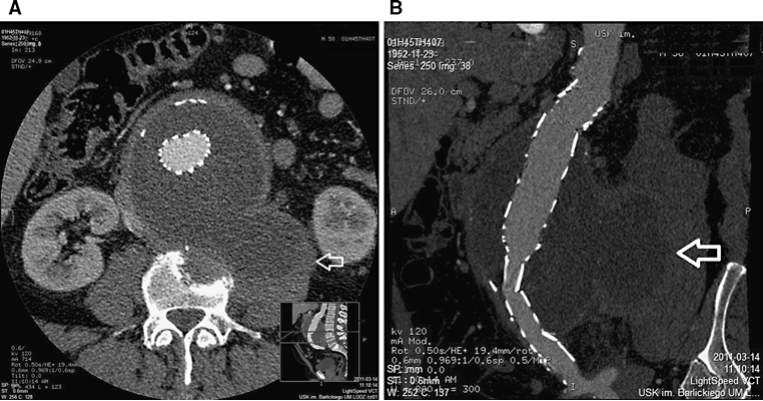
Angio-CT axial scan of aortic aneurysm below renal arteries. After implantation of the stent graft, patency is maintained. A Within the anterior and lateral walls neighboring the aorta, there is a soft tissue infiltration encompassing the inferior vena cava. A hematoma and inflammatory infiltration is probably present within the left iliopsoas muscle (*arrow*). Osteolysis of the L4 vertebral body is evident. B Reconstruction in the longitudinal plane confirms patency of the graft without leaking into the aneurysmal sac. The hematoma and inflammatory process have infiltrated the area of the iliopsoas muscle (*arrow*)
